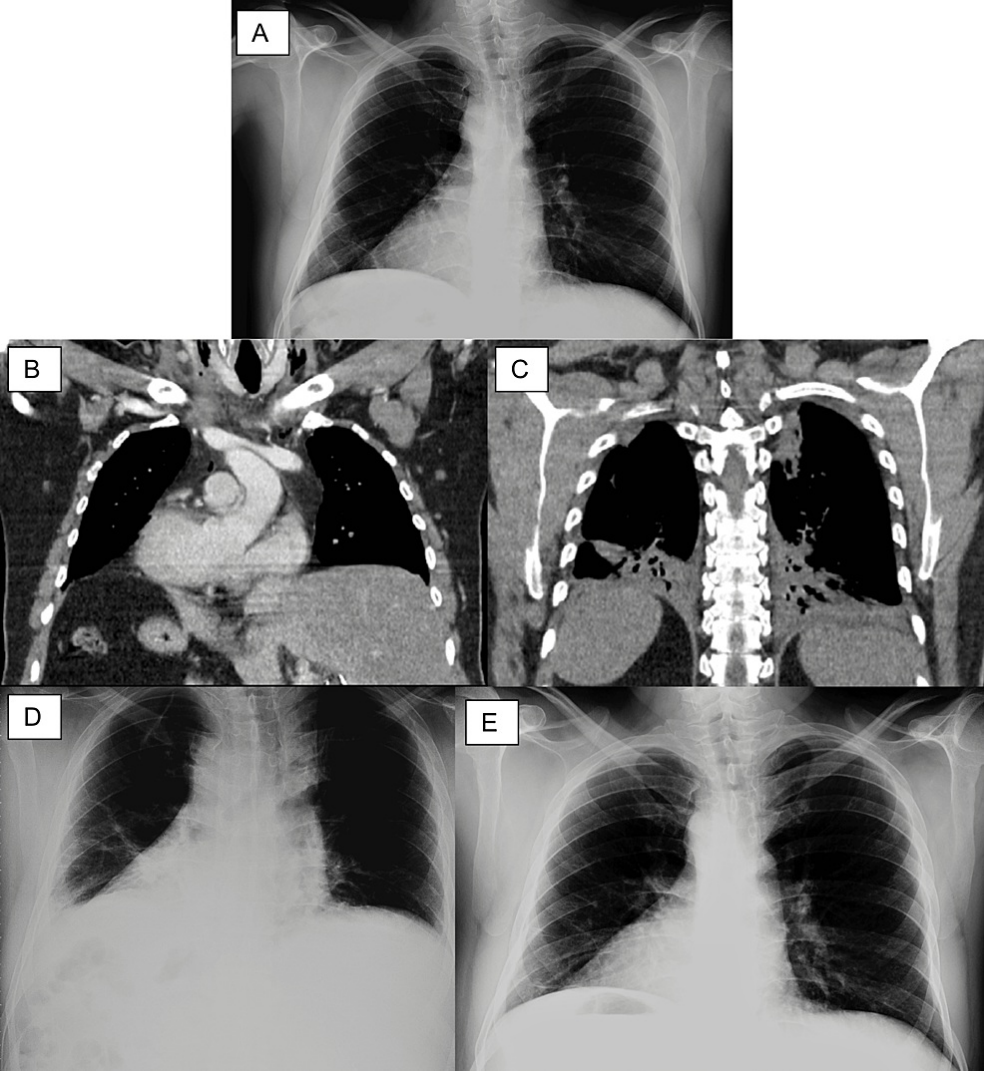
Pre and postoperative chest x-rays and simple and contrast-enhanced thoracoabdominal CT with postoperative pulmonary alterations. A) Preoperative chest x-ray that shows dextrocardia; B) Simple and contrast-enhanced thoracoabdominal CT with postoperative pulmonary alterations. Coronal section with a middle mediastinal window showing dextrocardia, with the liver situated in the left hypochondrium and the stomach in the right hypochondrium, this confirms the diagnosis, not only of situs inversus, but of SIT, as a complete transposition of thoracoabdominal organs is shown; C) Same CT scan, in a posterior coronal section showing the presence of incipient bilateral pleural effusion, bilateral posterobasal consolidations and basal atelectasis; D) Postoperative chest x-ray 48 hours after conservative management with respiratory exercises and oxygen therapy, showing a significant radiographic improvement with persistent right pleural effusion; E) Follow-up chest x-ray demonstrating resolution of atelectasis and pleural effusion. CT: computed tomography
